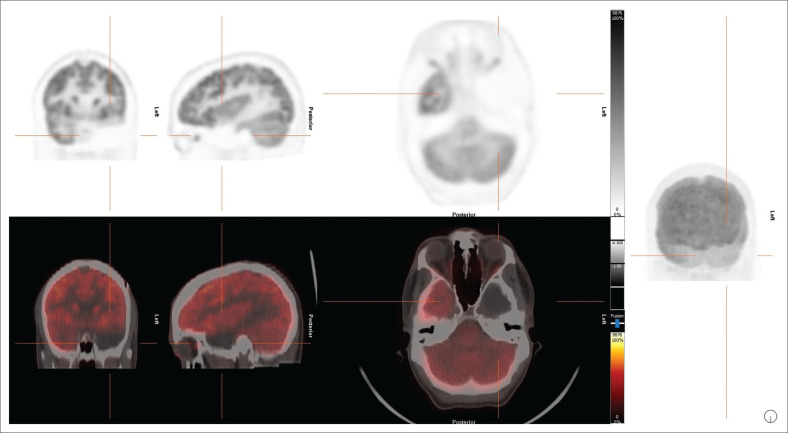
Interictal FDG PET scan in a patient with intractable temporal lobe epilepsy being worked up for epilepsy surgery. The scan demonstrates marked hypometabolism in the left temporal lobe, confirming lateralisation of the epileptogenic focus. The right panel demonstrates the PET maximum intensity projection; top row - PET orthogonal slices; bottom row - fused (PET-CT) orthogonal slices.
